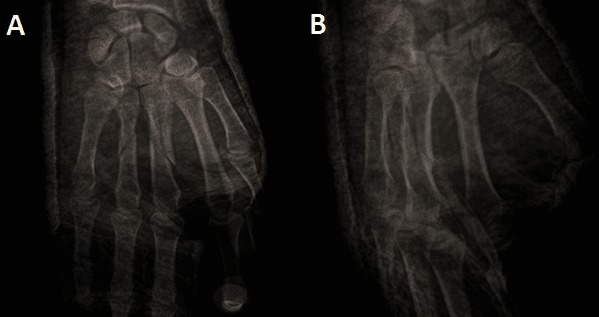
Plain view radiograph, Fig. 1B Oblique view radiograph. Both showing spiral and oblique fracture
